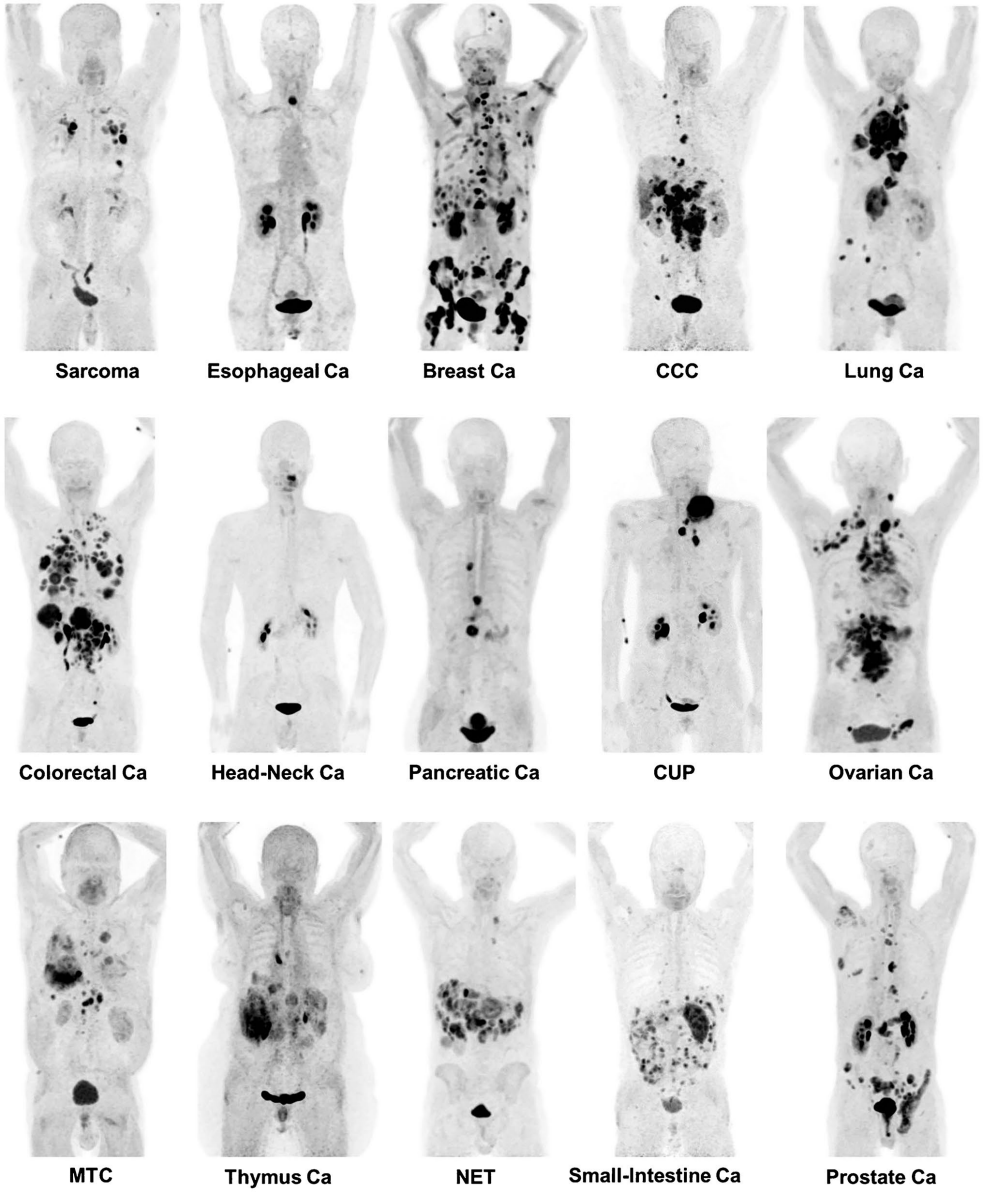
[^68^Ga]Ga-FAPI-04 PET/CT scans in 15 different cancer types demonstrating the pan-cancer potential of FAP-targeting radionuclide imaging. *Ca* cancer; *CCC* clear cell carcinoma; *CUP* carcinoma of unknown primary; *MTC* medullary thyroid cancer; *NET* neuroendocrine tumor *NSCLC* non-small cell lung cancer. The figure was originally published in JNM. Kratochwil et al. [95]. ^68^Ga-FAPI PET/CT: Tracer Uptake in 28 Different Kinds of Cancer (2019) Vol. 60(6): 801–805
